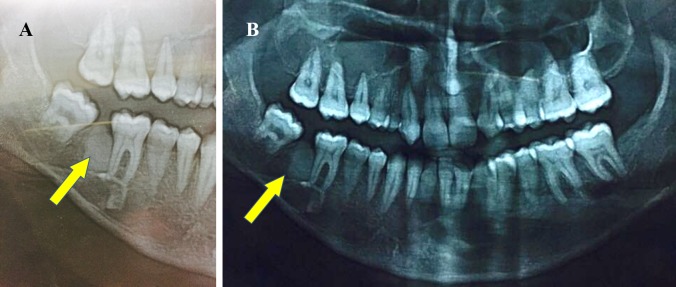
Orthopantomograph showing odontoma in the 47 region
